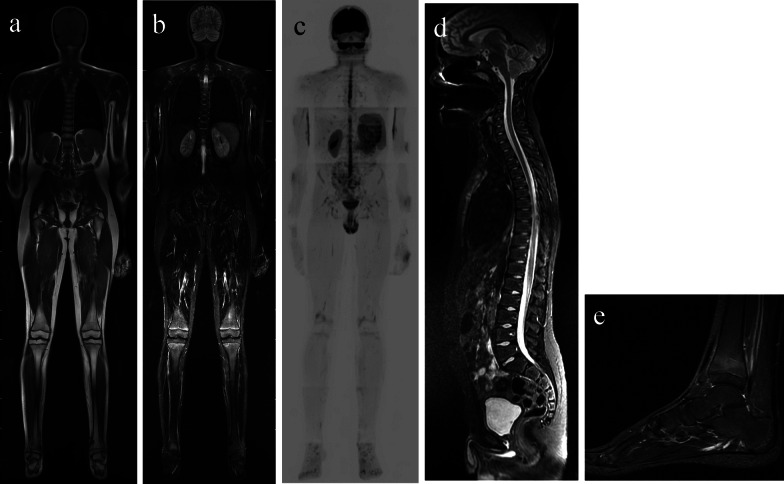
MRI protocol. Coronal T1 (a), coronal STIR (b), reconstructed coronal DWI inverted grayscale (c), sagittal STIR (d) of the whole spine and sagittal STIR of the feet (e)
